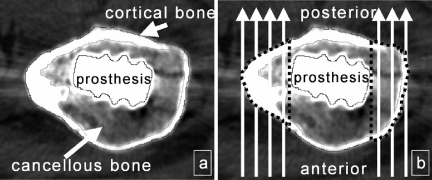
Separate circumferential evaluation of cortical and cancellous BD (mg CaHA/mL) with CT-assisted osteodensitometry (a) compared to the analysis of single zones of interest (dotted lines) in the AP view with DXA (b).
